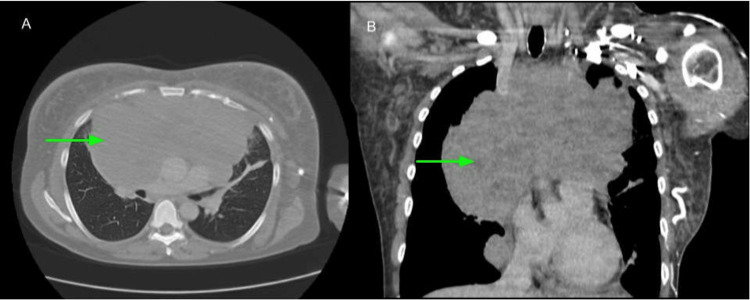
CT scan of the chest with contrast (A: axial view; B: coronal view) showed a large anterior mediastinal mass (green arrows) extending to both hilar regions.
